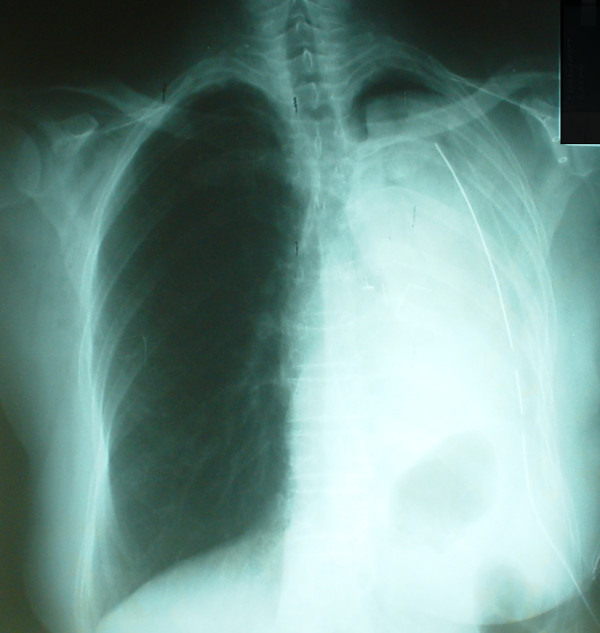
Chest X-ray 10 hours later with consolidation and loss of air entry.
